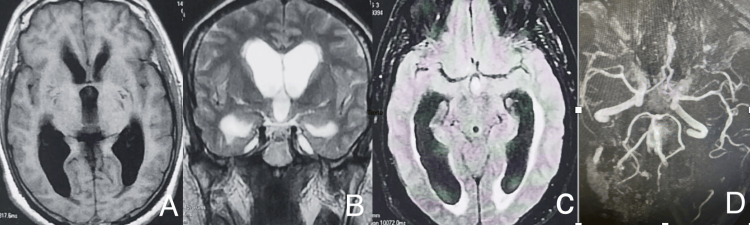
Brain MRI: (A) T1-weighted image axial plane, (B) T2-weighted image coronal plane, (C) fluid-attenuated inversion recovery sequence axial plane, and (D) MR angiography sequences. (A-C) Subarachnoid hemorrhage with intraventricular flooding in the third ventricle associated with an acute moderate active triventricular hydrocephalus. (D) MR angiography sequences showing no abnormalities in the circle of Willis.
